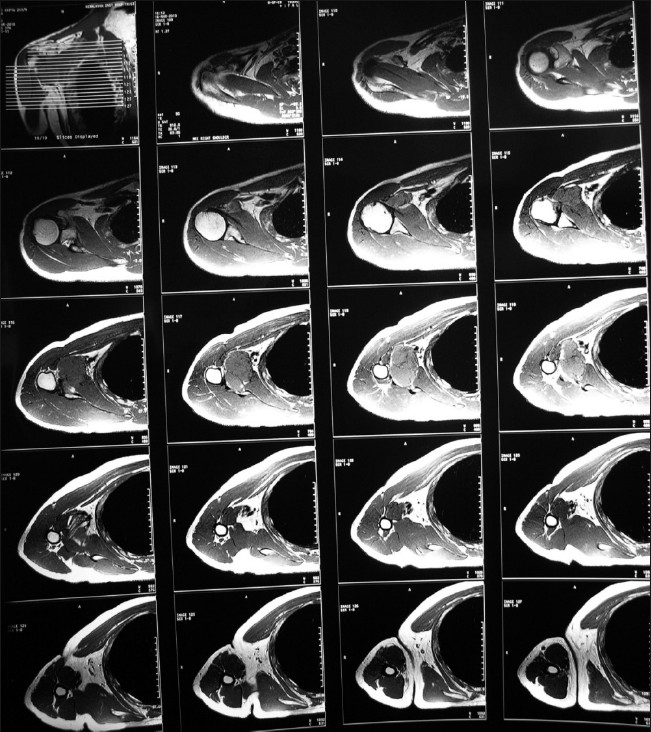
Magnetic resonance images showing the extent and location of the tumor. Note its relation with the subscapularis and the glenoid
